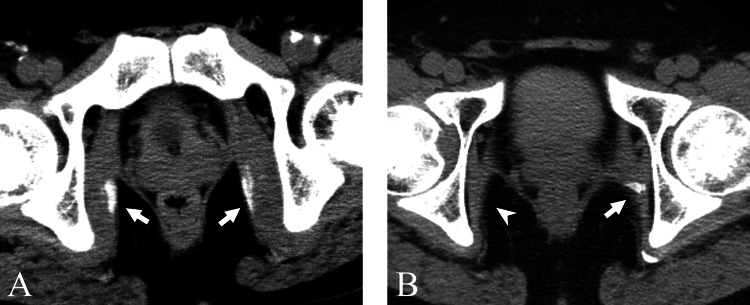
Case four A 55-year-old man with myositis ossificans in the left short external rotator muscles. (A) On the 24th day of hospitalization, CT showed swelling and calcification in the muscles bilaterally (arrows). Manual muscle testing score in both lower limbs was 2. (B) On the 360th day after admission, follow-up CT was performed due to suspected lumboperitoneal shunt failure. On the left side, ossification progression was observed (arrow). On the right side, both swelling and calcification resolved without ossification (arrowhead).
